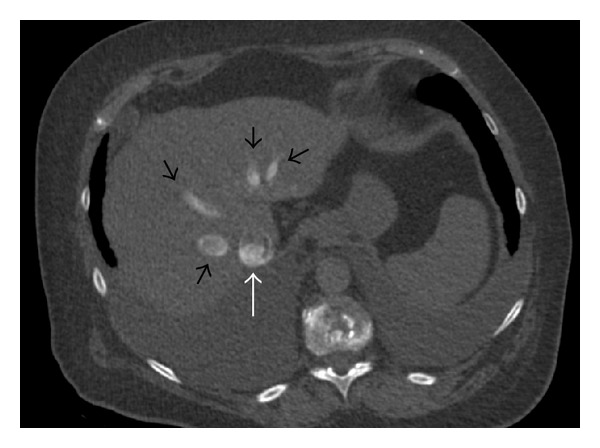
Computed tomography showing significant (grade 5) reflux of contrast media in the inferior vena cava (white arrow) and hepatic veins (black arrows) seen in a 75-year-old man diagnosed with pulmonary embolism and right-ventricular dysfunction.
